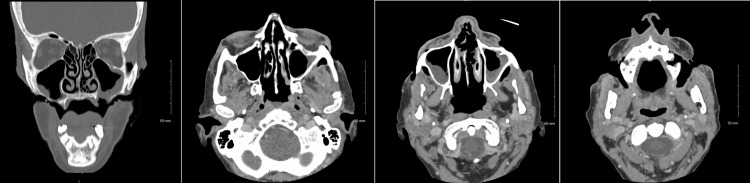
De-identified Patient's CT Imaging Coronal and transverse CT imaging of patient's disease.
